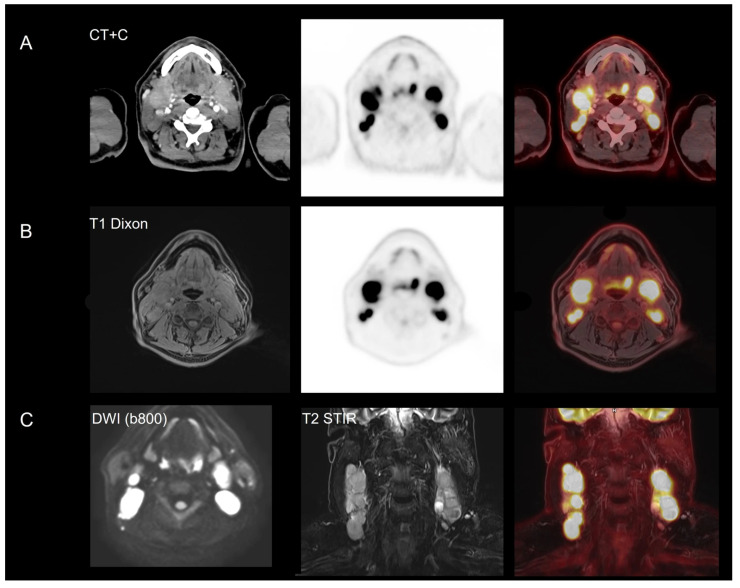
Regional focused imaging—head/neck. Patient with a swelling on both sides of the neck, but no clinical signs of a primary tumor. Both PET/CT (A) and BIOGRAPH One PET/MRI (B) revealed the primary tumor in the base of the tongue, in addition to metabolically active lymph nodes on both sides of the neck. Biopsy confirmed a tongue base cancer, squamous cell carcinoma, T2N3M0, HPV+. In the lower row (C), DWI shows concordant diffusion restriction corresponding to both [^18^F]FDG avid lymph nodes and the primary tumor. Coronal T2 STIR and [^18^F]FDG show enlarged metastatic cervical lymph nodes. PET acquisition was performed using continuous bed motion at 1.5 mm/s, corresponding to approximately 3 min per field of view (FOV) for PET/CT, compared to 20 min for a single-bed PET/MRI acquisition.
